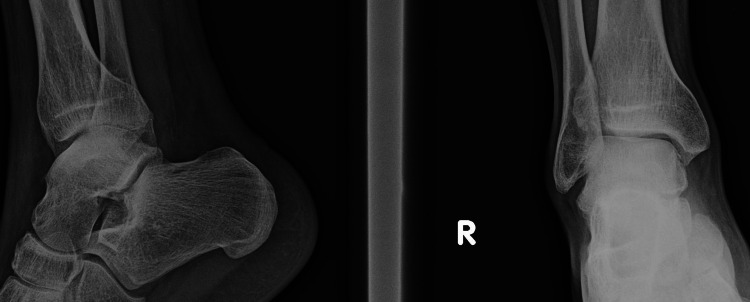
Plain radiographs a year after the excision. The patient has fully recuperated and returned uneventfully to his daily activities and amateur sports.
